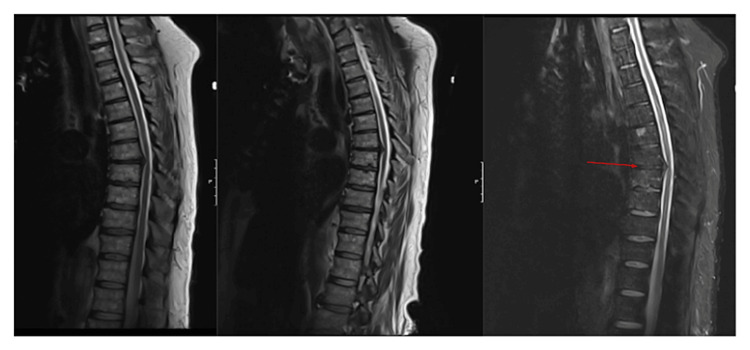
MRI of the thoracic spine in flexion T2-sequences of a MRI thoracic spine without contrast in a neutral (left) and with the patient in flexion (center) showing no dynamic compression on the spinal cord from the T7-8 disc herniation. Short T1 inversion recovery (STIR) sequences from this MRI (right) additionally show no edema within the intervertebral disc to suggest an acute herniation (red arrow).
